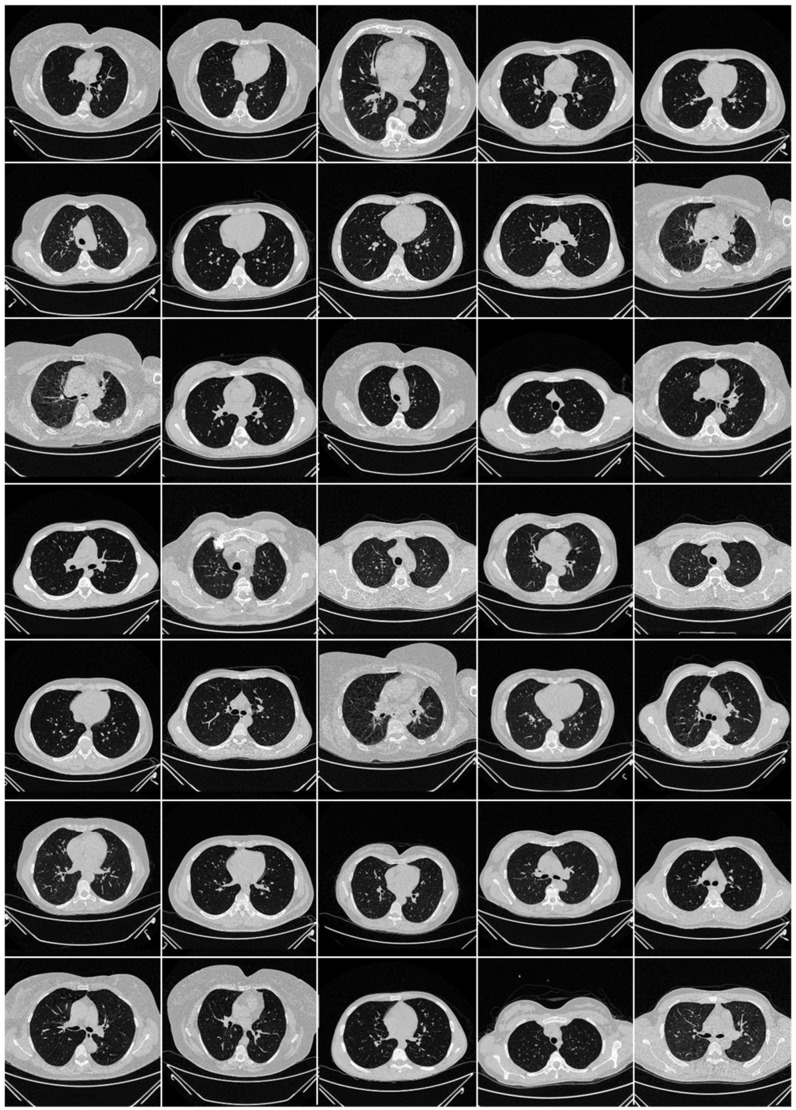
Raw control CT slice taken from Italian data set.
